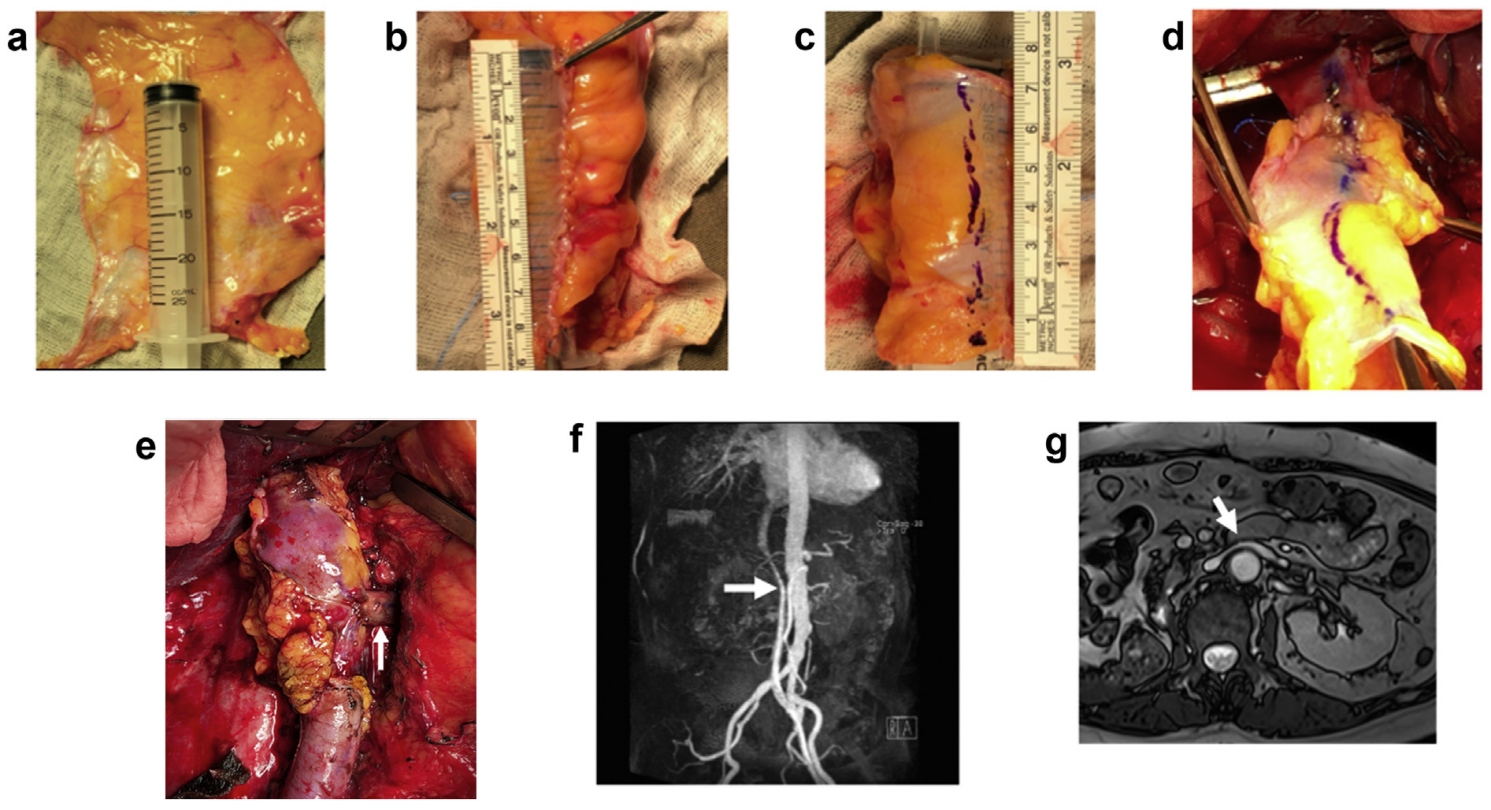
a, Wrapping of falciform ligament around a 20-mL syringe. b, The edges of the falciform ligament were sutured using 4-0 Prolene suture, and excess adipose tissue was trimmed off. c, Marking of the longitudinal alignment line of tube graft. d, Anastomoses of the interposition tube graft to the inferior vena cava (IVC). e, Implantation of the left renal vein (*arrow*) onto the interposition tube graft. f, Postoperative magnetic resonance imaging scan at 12 months showing patent falciform ligament tube graft (*arrow*). g, Patent left renal vein (*arrow*) on 12-month postoperative magnetic resonance imaging scan.
